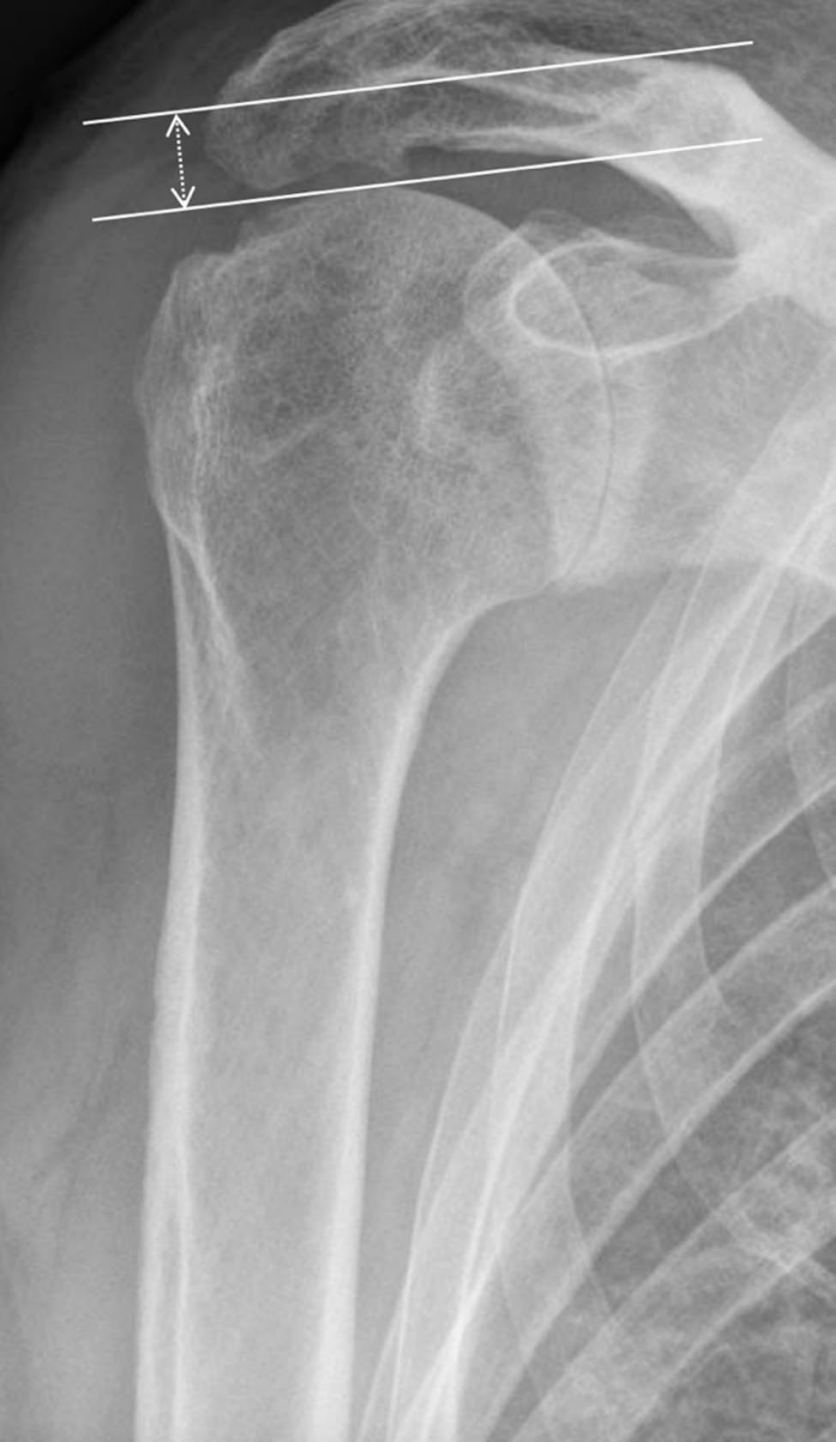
Measurement of the acromiohumeral distance (AHD) on a standardized anteroposterior radiograph, defined as the perpendicular distance between the undersurface of the lateral acromion and the superior border of the greater tuberosity
